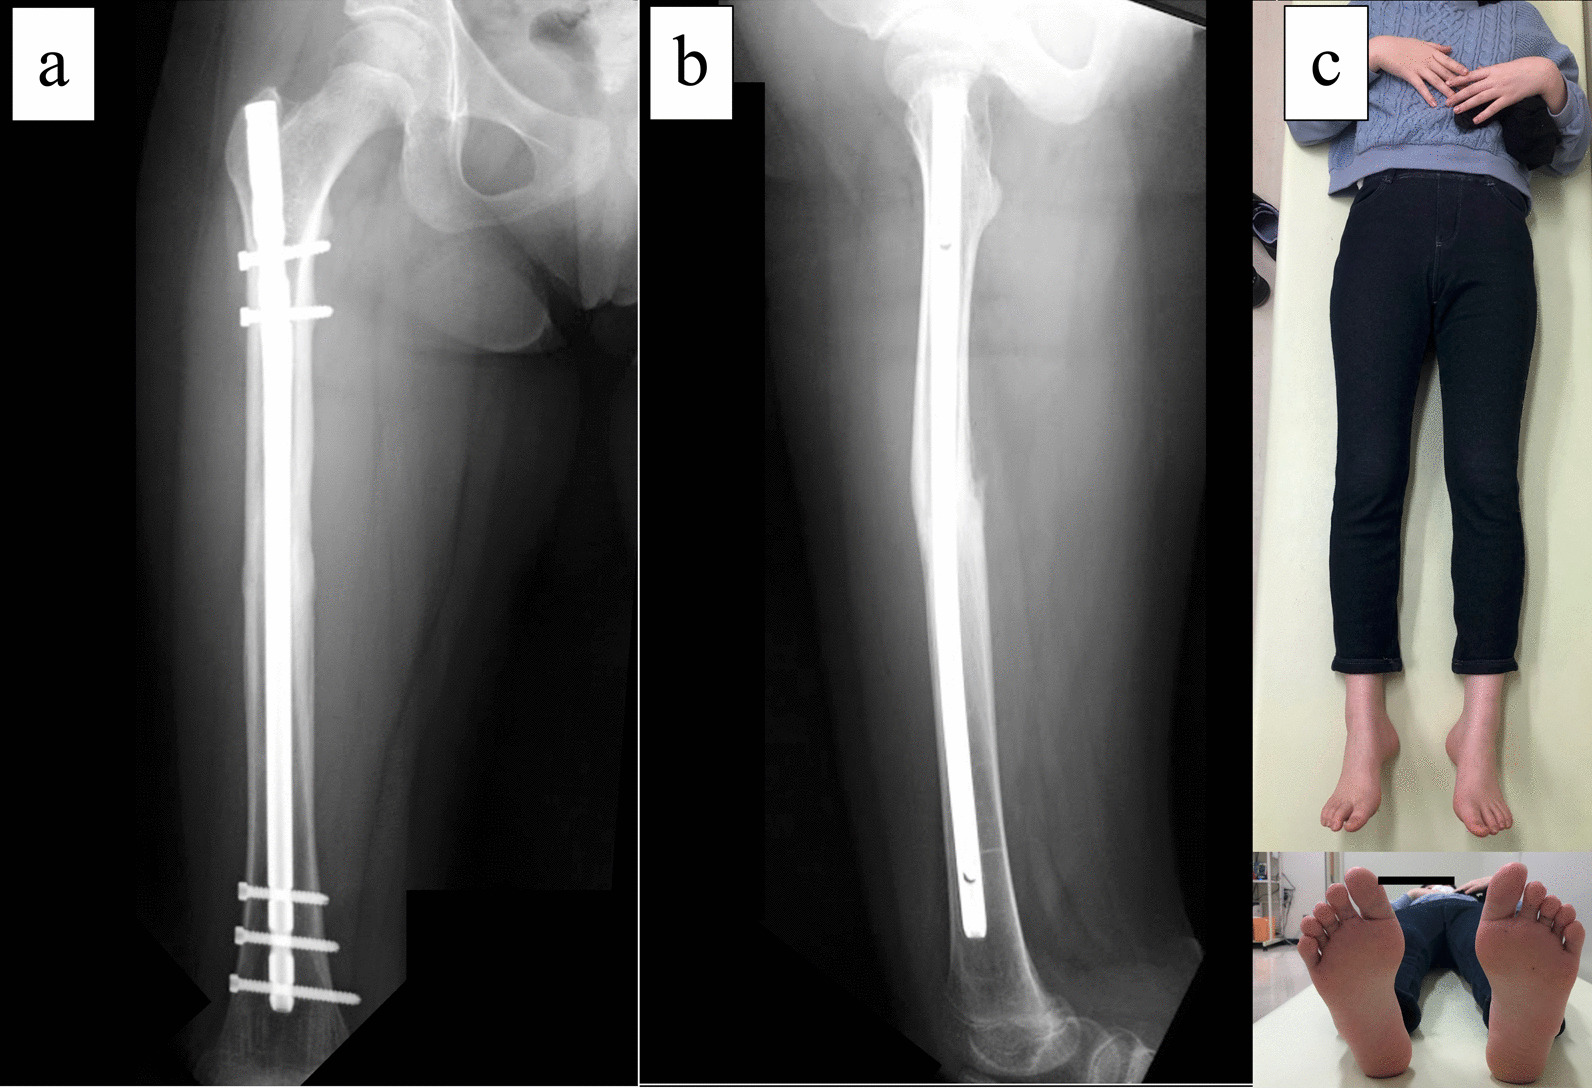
X-ray images and external views of the patient’s lower extremity 10 months after revision surgery. a Anteroposterior image, b lateral image, c photograph of both lower extremities, and d photograph of both feet viewed from the caudal side. There is no leg length discrepancy or rotational deformity
